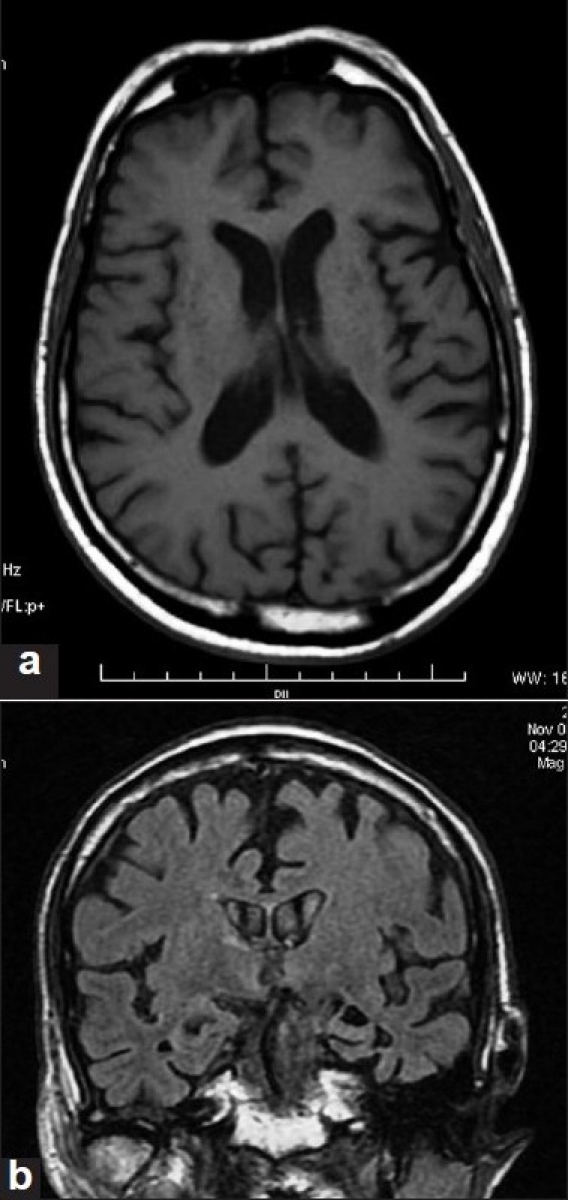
Magnetic resonance imaging brain T1W axial (a) and coronal (b) images showing left perisylvian and temporal lobe atrophy in a 77-yearr-old patient with progressive nonfluent aphasia
